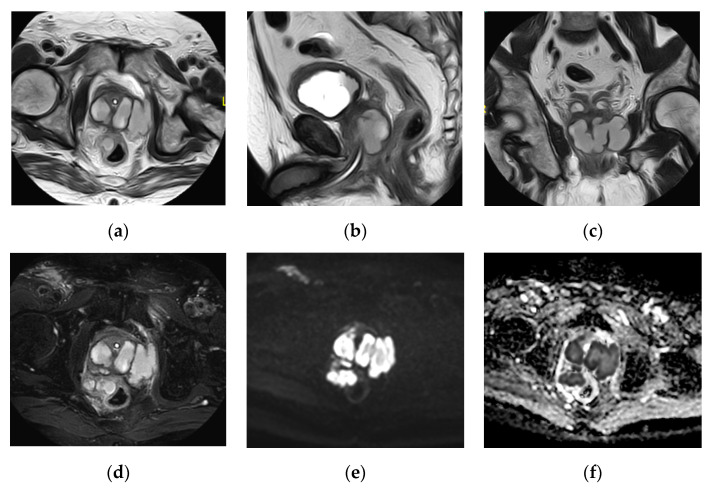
Prostatic abscess. (a) Axial, (b) sagittal, (c) coronal T2 weighted images and (d) Axial T2 weighted image with fat suppression show that the enlarged prostate is a seat of a multilocular cystic lesion with thick wall and thick septae, displaying fluid signal intensity, showing extraprostatic extension with periprostatic fat stranding and inflammatory reaction, it is seen indenting the right lateral wall of the rectum, and extends to the left obturator internus muscle. The DWI (e) and apparent diffusion coefficient (ADC) map (f) show the abscess as an area of restricted diffusion; high signal intensity (SI) at DWI and low SI at ADC map.
